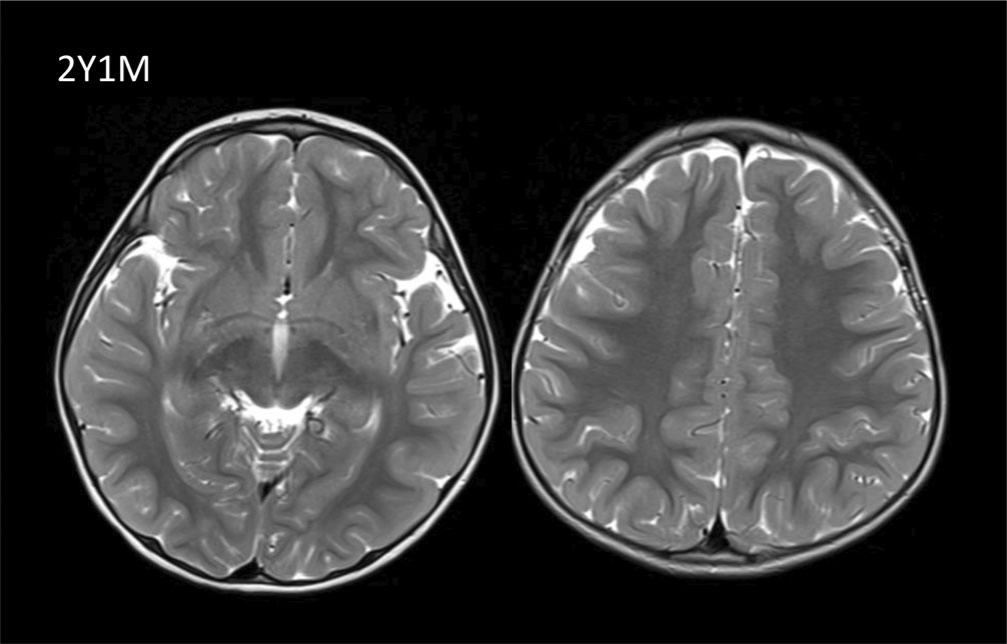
Neuroimaging of patient 2. Normal MRI at 2 years of age.
